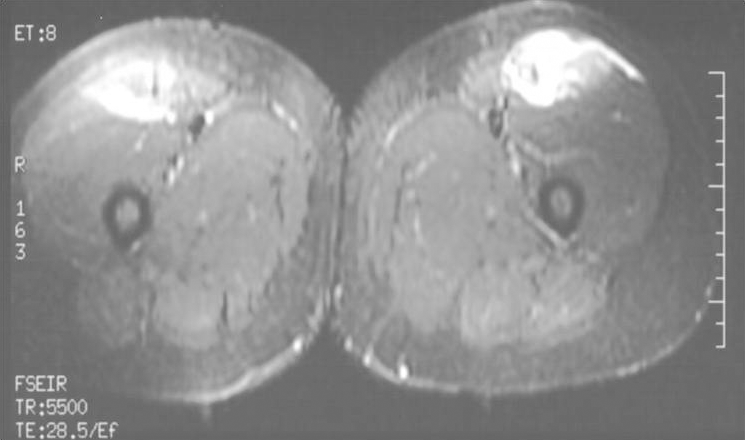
Horizontal MRI section imaging the haematoma on both thighs.
